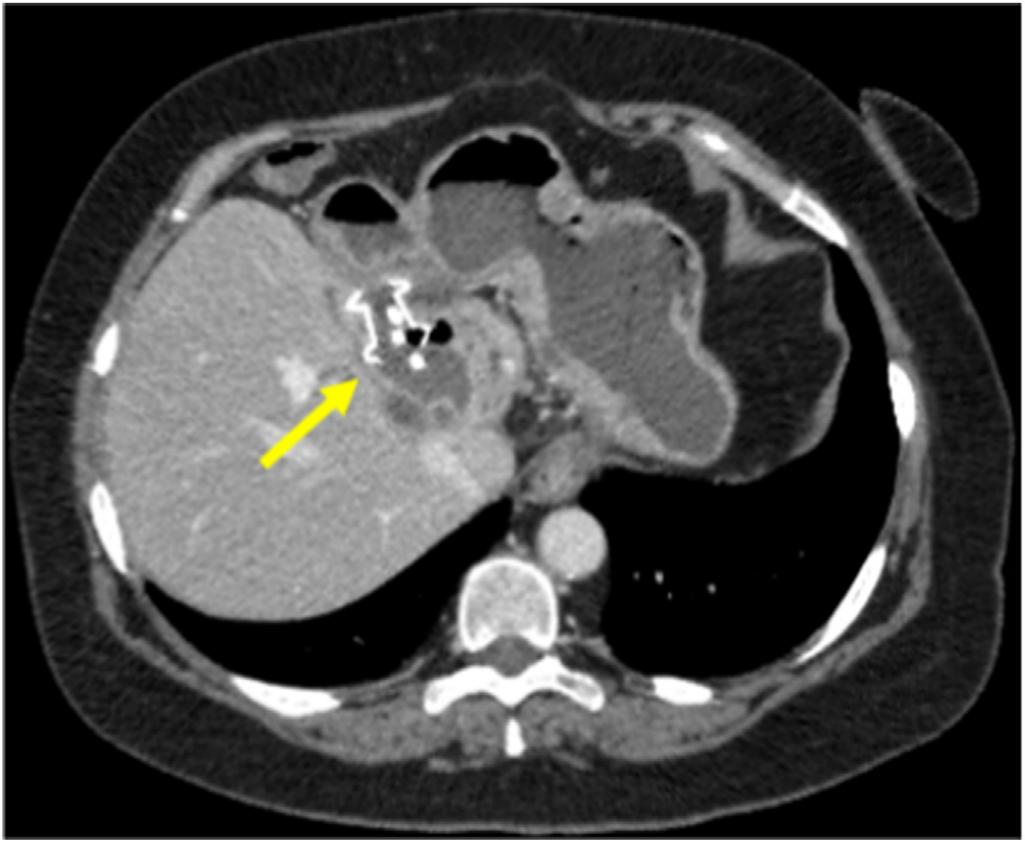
A CT scan showing an undrained portion of the cyst concerning for an abscess.
